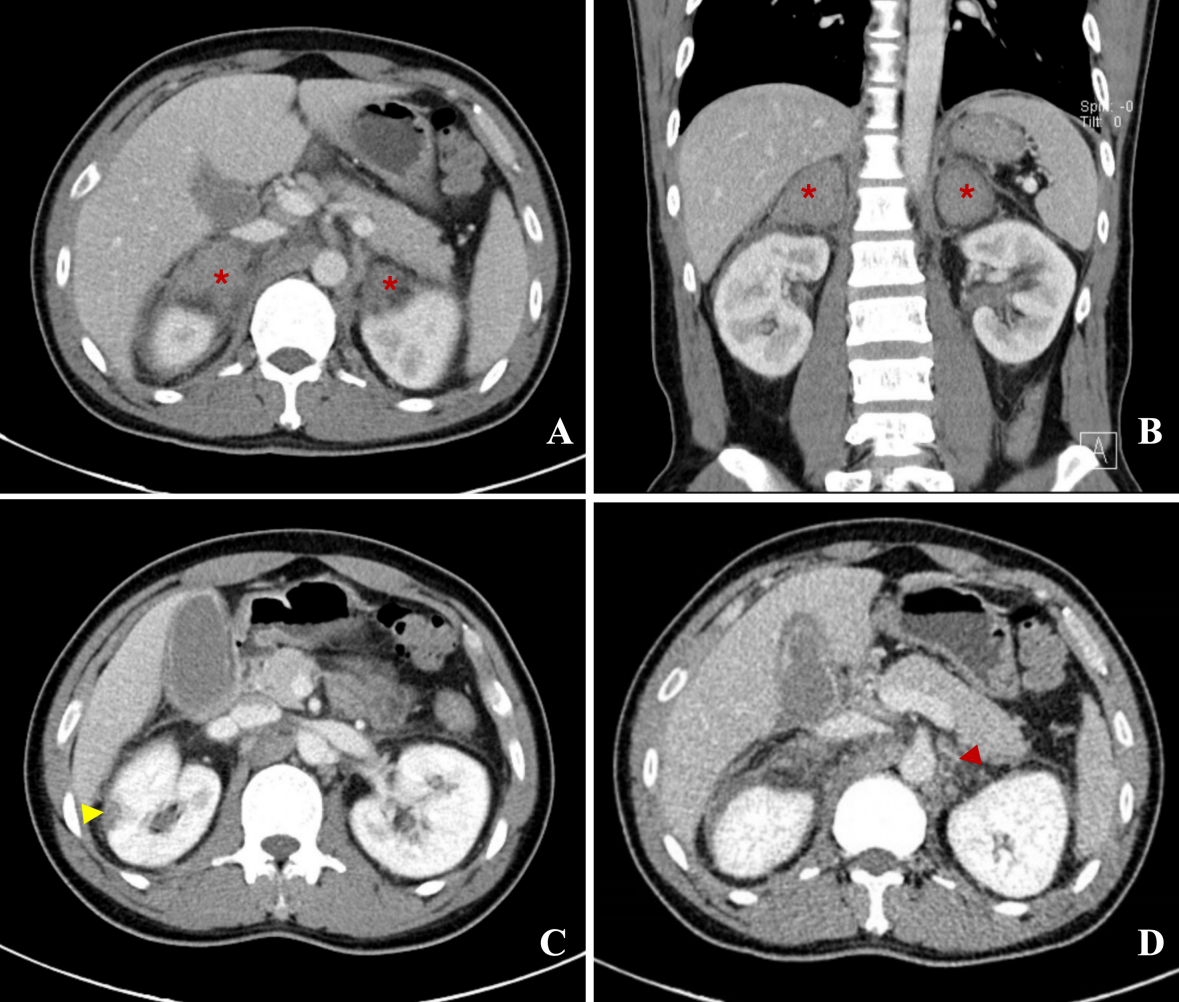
A, B: Transverse and coronal section of enhanced abdominal tomography (CT), showed clearly bilateral adrenal hemorrhage (AH), manifesting as slightly high-density shadow with no enhancement, marked with asterisk. C: A wedge-shaped hypo-enhanced lesion indicating ischemic change was seen at marginal of right kidney, marked with yellow arrow. D: A patchy low-density shadow was found in left adrenal vein, marked by red arrow, which might be a thrombosis.
